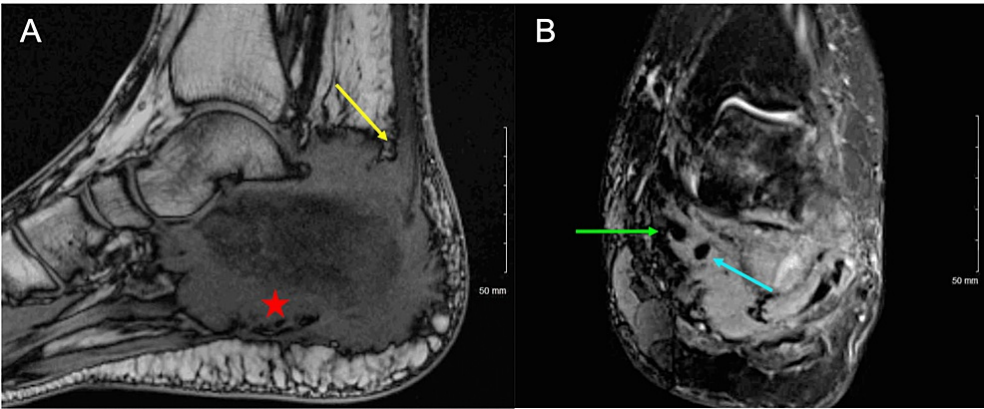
Multisequence, multiplanar left ankle magnetic resonance imaging without contrast. (A) Sagittal T1 image showing a circumferential soft tissue mass of the calcaneus with irregular cortical disruption. There is also involvement of the Achilles tendon insertion (yellow arrow) and posterior plantar fascia (red star). (B) Coronal short tau inversion recovery image showing the mass to completely encase the flexor hallucis longus tendon (blue arrow). The mass also abuts the deep margins of the flexor digitorum longus tendon (green arrow).
